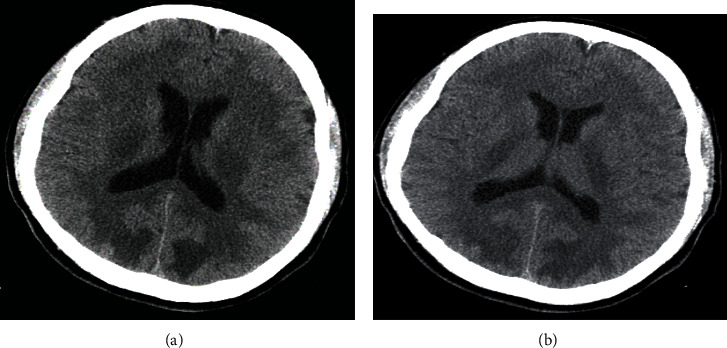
(a, b) Computed tomography scan of the brain without contrast, showing white matter disease in both cerebral hemispheres and cerebellum.
